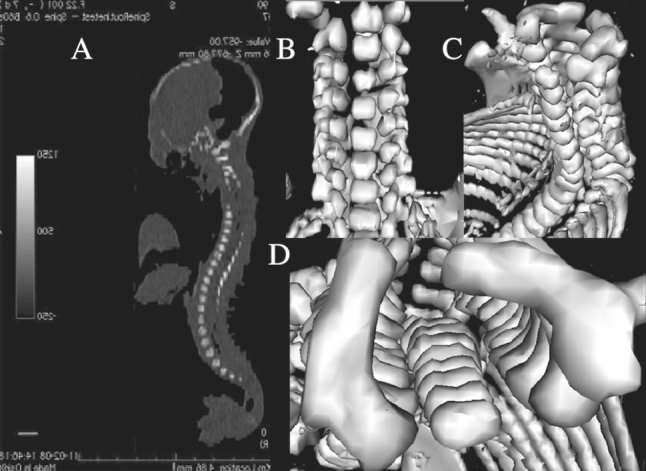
CT of a female fetus aged 25 weeks recorded in DICOM formats (a) and assessed by Osirix 3.9 in frontal (b), lateral (c), and horizontal (d) planes
